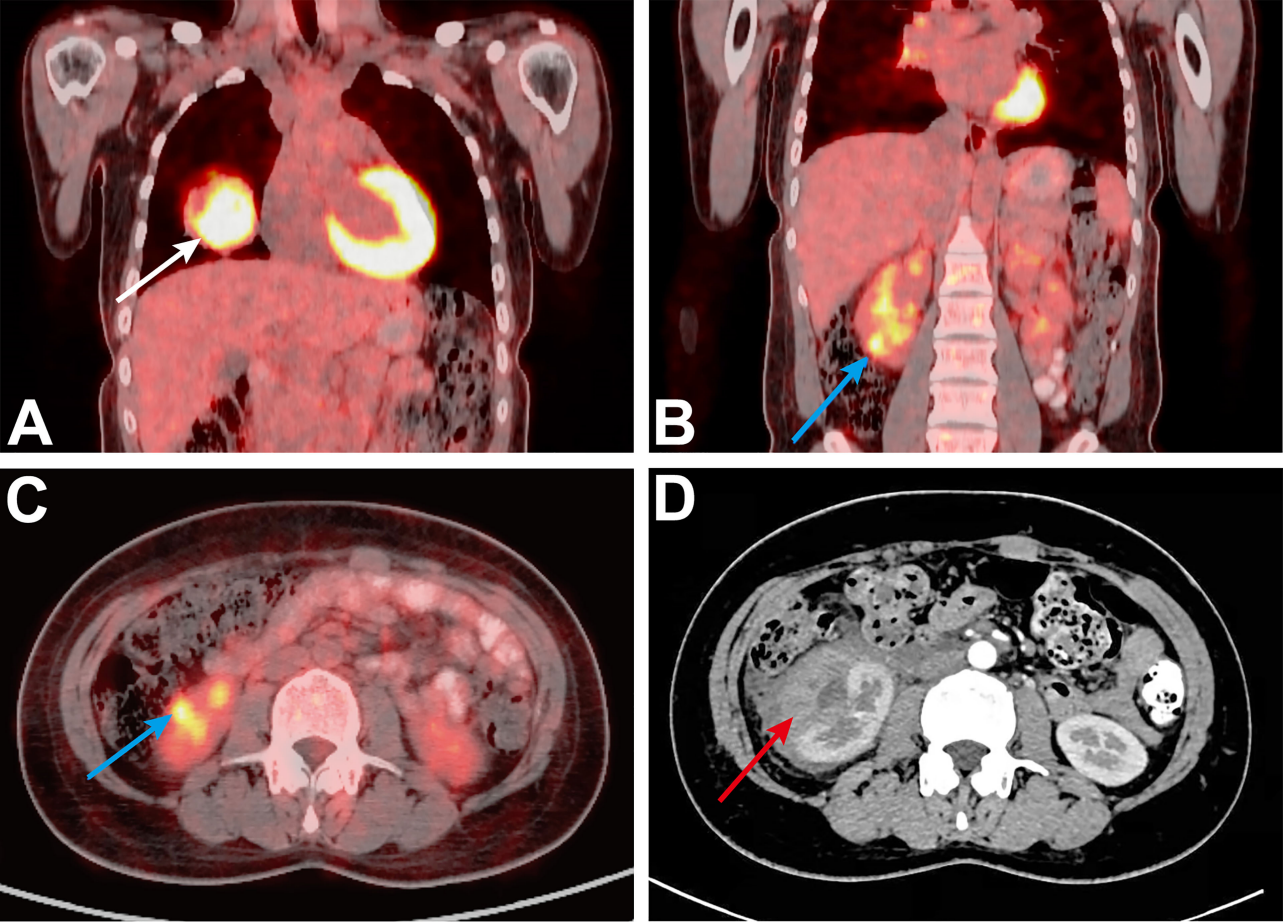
(A) Initial PET/CT, showing the right lung mass (solid white arrow). (B, C) Initial PET/CT, showing the mass of the lower pole of the right kidney (SUV_max_ = 8.7, solid blue arrow). (D) Preoperative CT shows a large subcapsular hematoma (solid red arrow).
